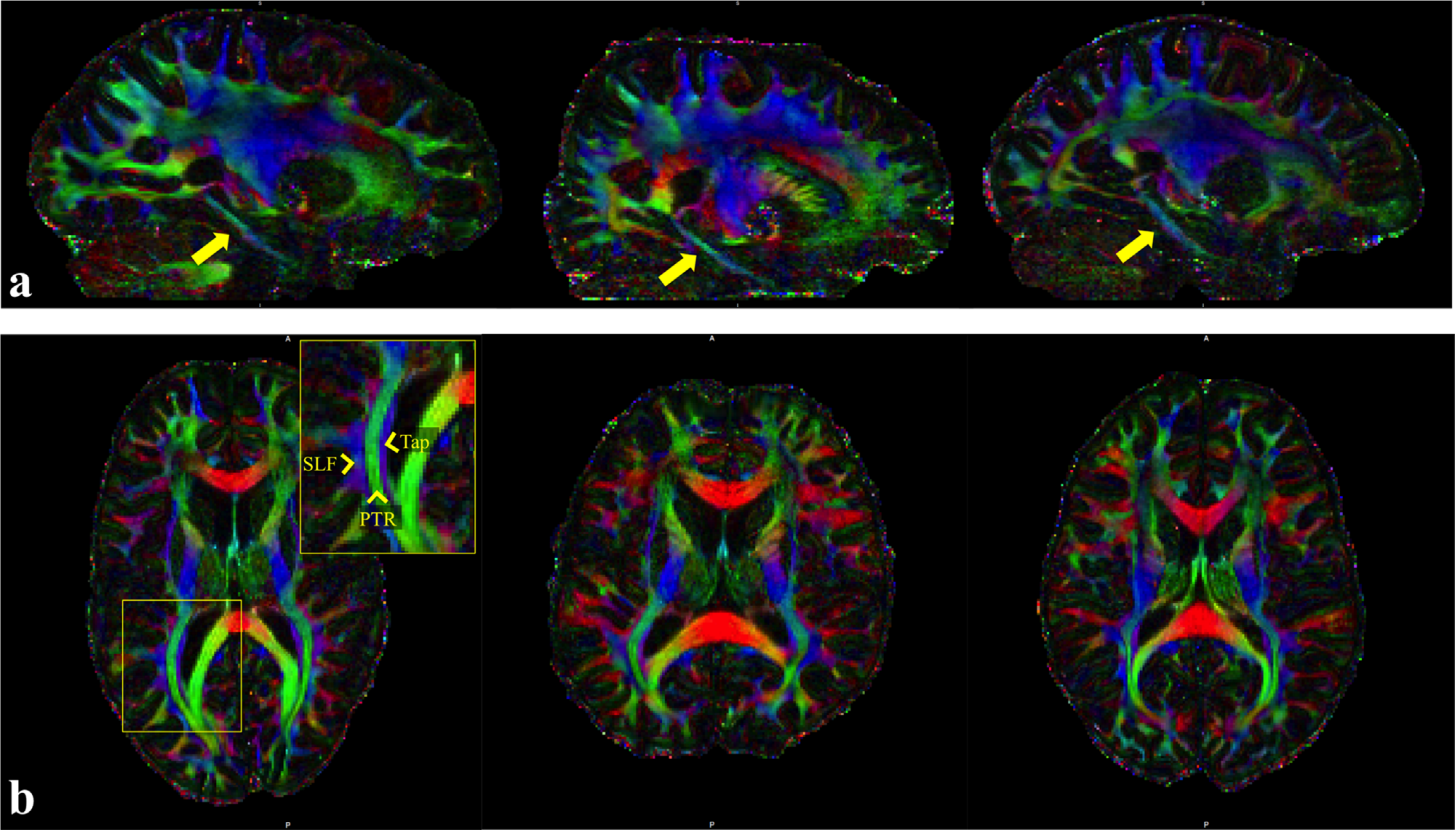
Colour-coded maps of the principle eigenvectors for the high resolution DTI data (red, right–left; green, anterior–posterior; blue, superior–inferior). The high spatial resolution enables (a) clear detection of thin fibre tracts such as the cingulum bundle descending into the temporal lobe (yellow arrows) and (b) crisp disambiguation of some fibres which can be difficult to separate using low resolution diffusion MRI (superior longitudinal fasciculus, SLF, posterior thalamic radiation, PTR, the tapetum of the corpus callosum, Tap). (For interpretation of the references to color in this figure legend, the reader is referred to the web version of this article.)
